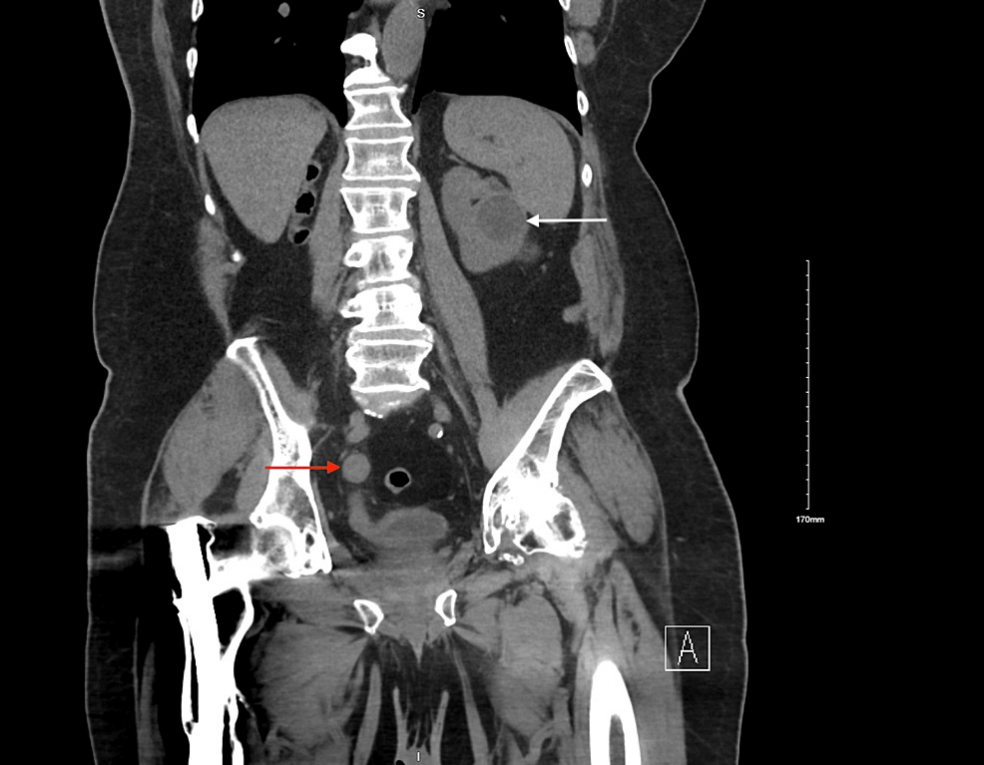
Abdominal and pelvic computed tomography (CT) showing right sided dilated ureter (red arrow) and a stable, left sided renal mass (white arrow).
